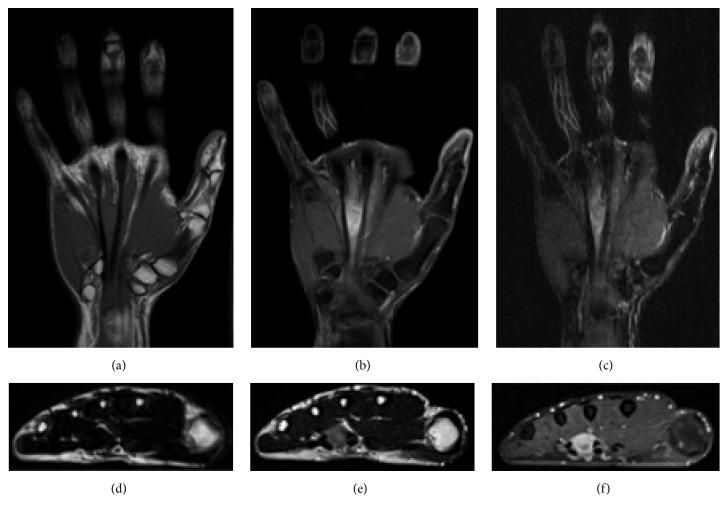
Magnetic resonance imaging revealed a 20 × 15 × 10 mm mass of low intensity on T1-weighted images (a, d) and high intensity on T2-weighted images (b, e) and gadolinium enhancement (c, f).
